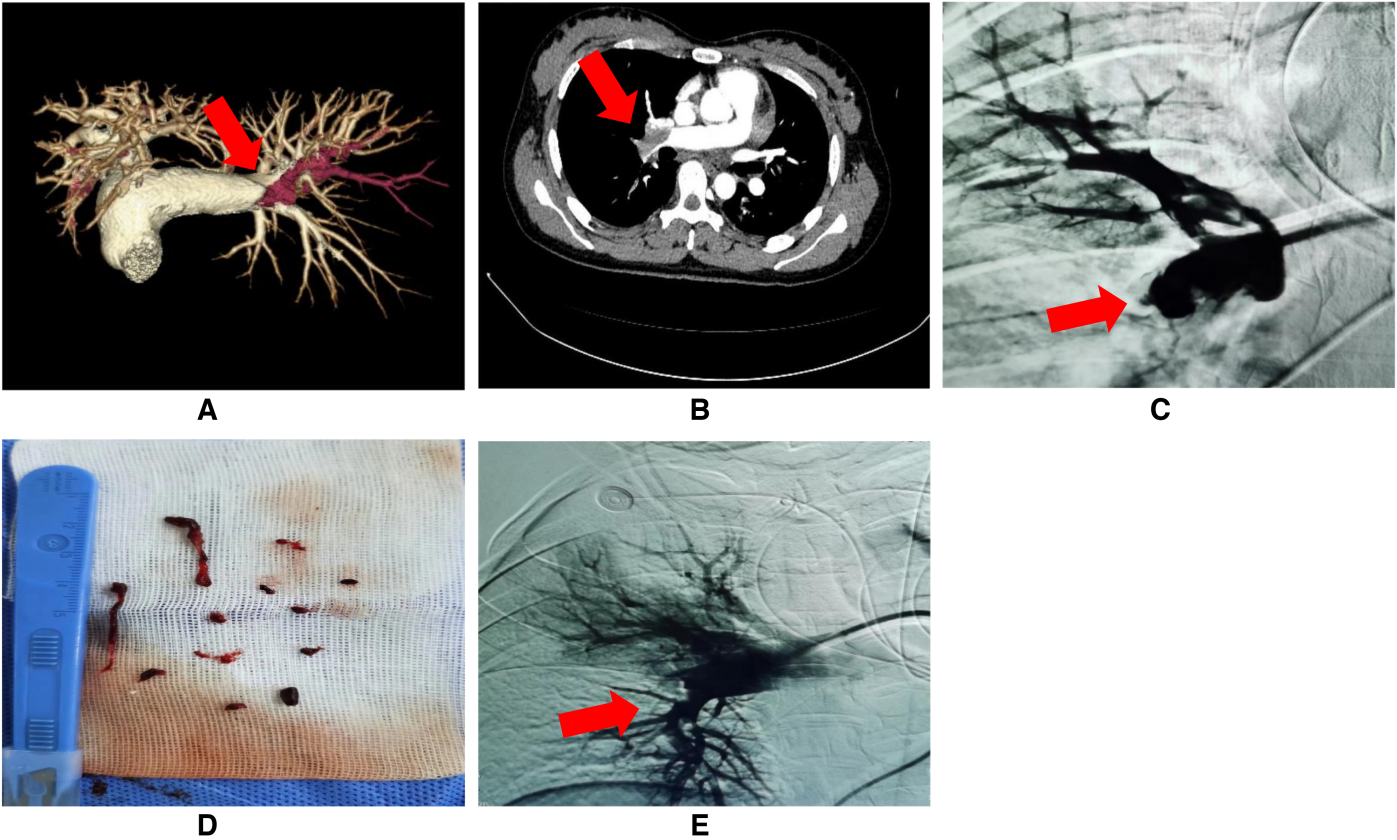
(A–E) Preoperative examination and interventional operation of PE. (A,B) Pulmonary embolism (PE) detected by preoperative CT angiography (red arrow: the site of PE); (C) PE confirmed by target pulmonary artery angiography (red arrow: the site of PE); (D) Successful thrombus aspiration; (E) Rechecked angiograms after interventional therapy (red arrow: the site of PE resolution).
